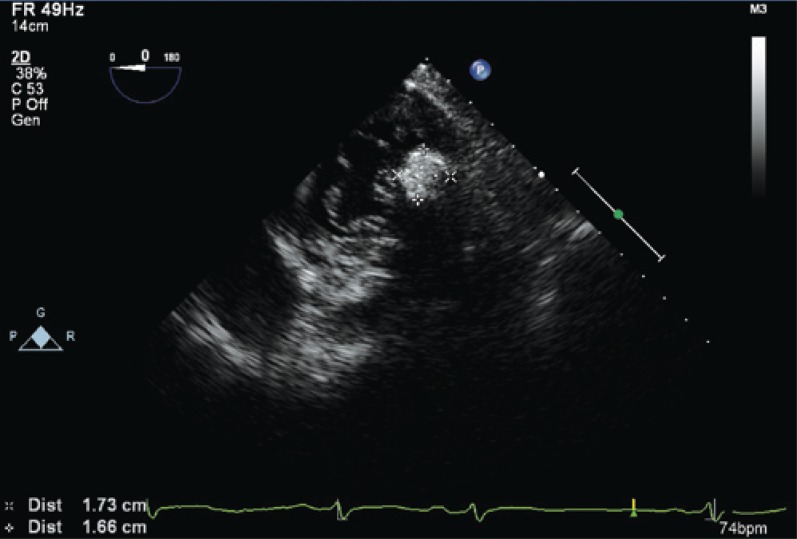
Transgastric view by transesophageal echocardiogram in mitral valve level showing the same mass located in the posterior mitral valve annulus close to P2 segment
